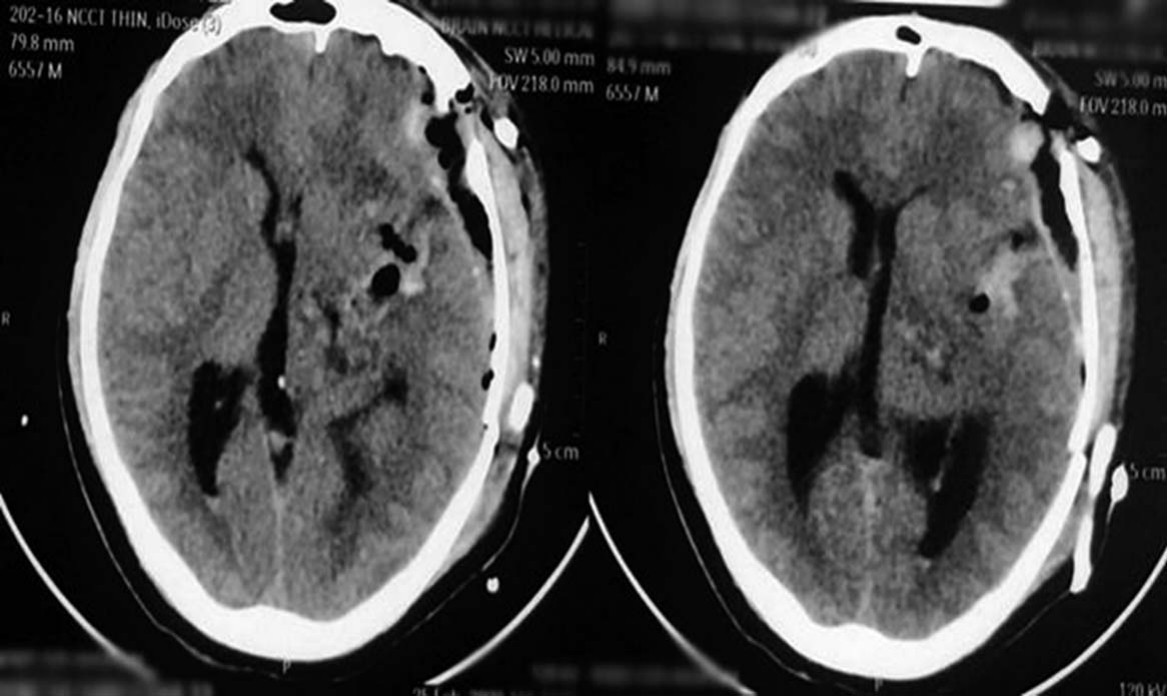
The postoperative noncontrast computed tomography images showing the total excision of the lesion.
